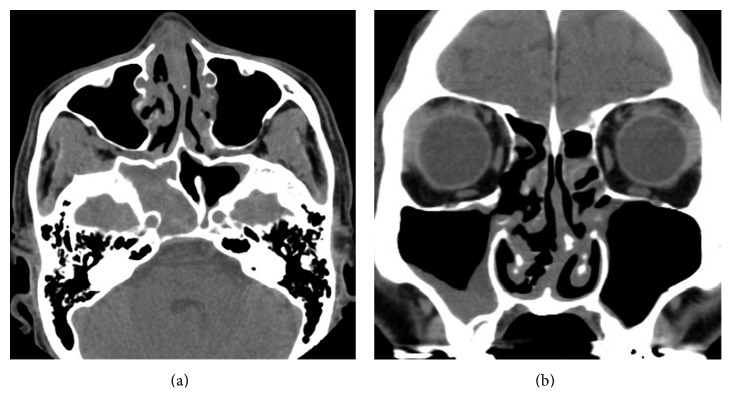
CT scan at first visit shows swelling of the mucosa in the bilateral ethmoid sinuses (a) along with a soft-tissue area occupying the right sphenoid sinus (b).
